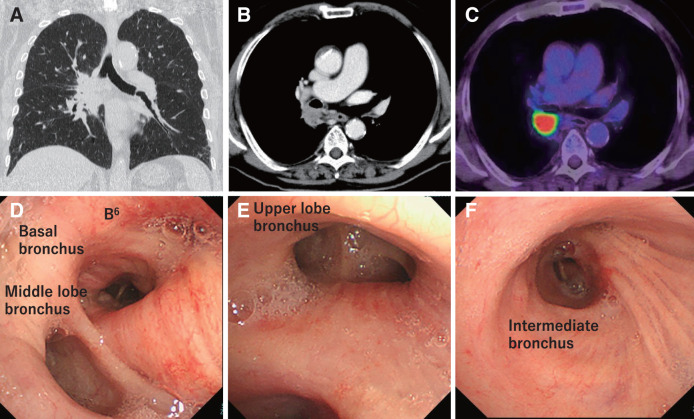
Imaging scan of the patient before chemoimmunotherapy. (A, B) Computed tomography scan showing a 51-mm mass in the right lower lobe, which extended to the dorsal side of the right upper lobe and hilar lymph node. (C): PET-CT showed tumor maximum standardized uptake value (SUVmax) was 13.3. (D–F) Bronchoscopy showing the occlusion of B6 and erythema of the bronchial mucosa on the dorsal surface of the upper lobe bronchus, intermediate bronchus, and lower lobe bronchus. PET-CT, positron emission tomography-computed tomography
